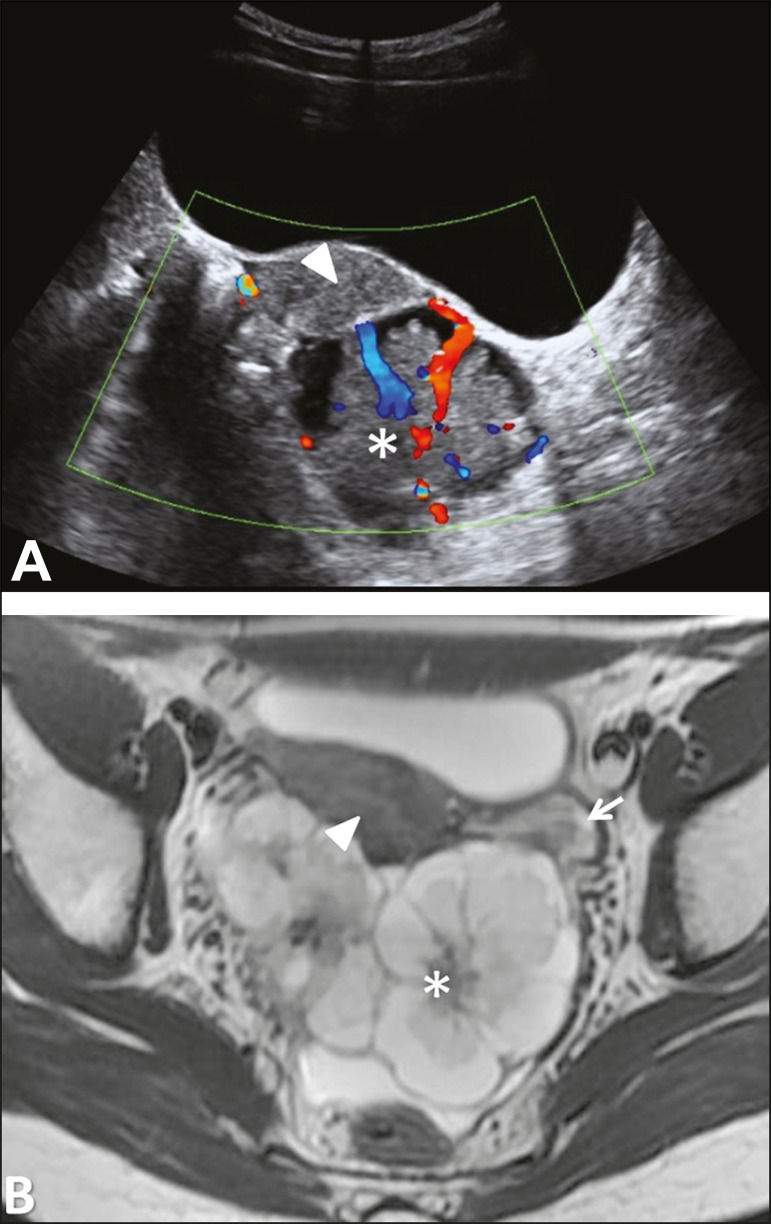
Papillary cystadenoma of the broad ligament in a 16-year-old patient with von Hippel–Lindau disease. A: Transvaginal ultrasound imaging showing a solid hypoechoic tumor with a cleavage plane with the uterus and color Doppler signal. B: Axial T2-weighted imaging demonstrating it as an intraperitoneal, retrouterine tumor, with mixed characteristics.
